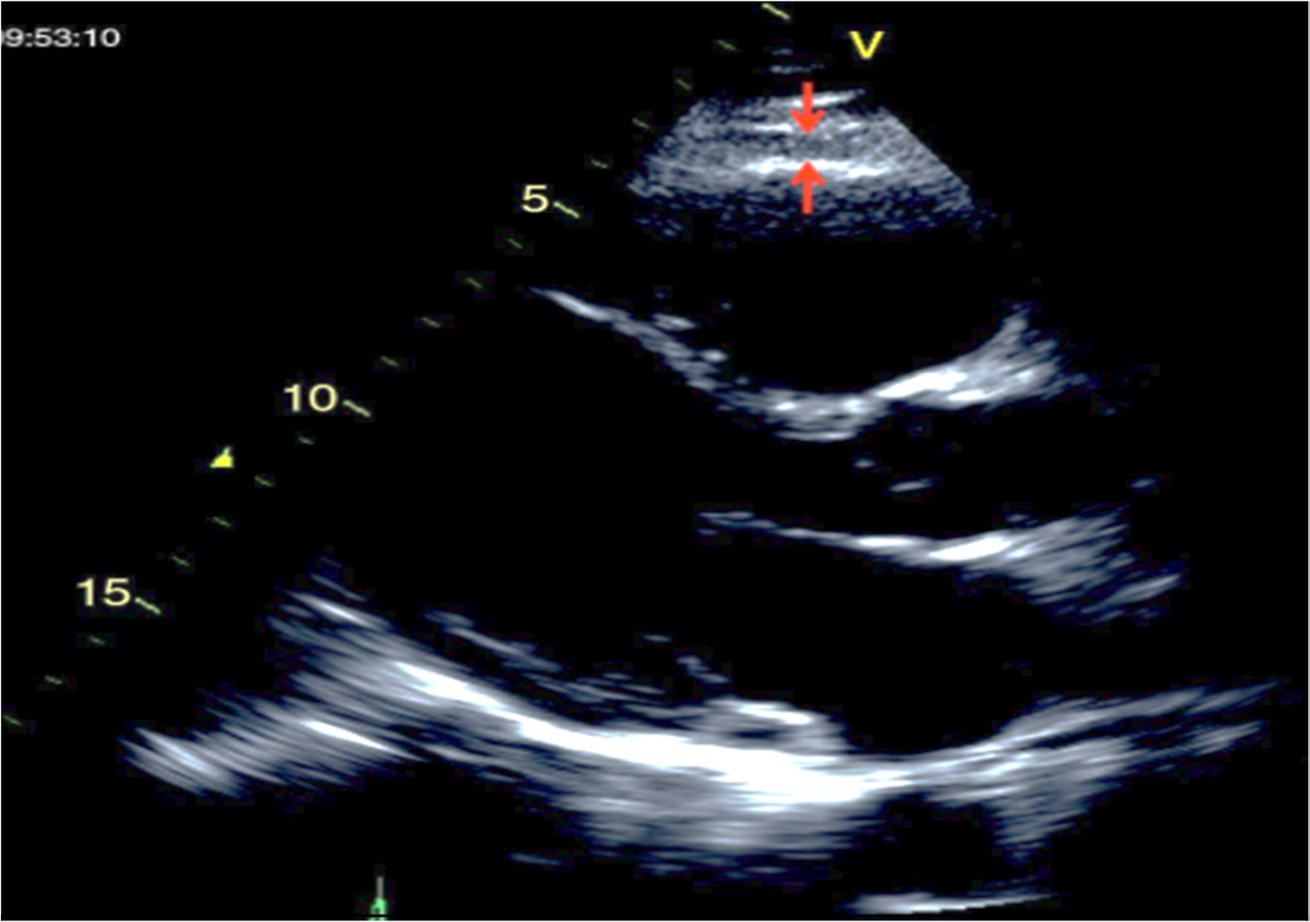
Epicardial Adipose Tissue Thickness. Echocardiographic measure of epicardial fat thickness (pointed by the red arrows) identified as the echofree space between the outer wall of the myocardium and the visceral layer of pericardium in the parasternal long-axis view
